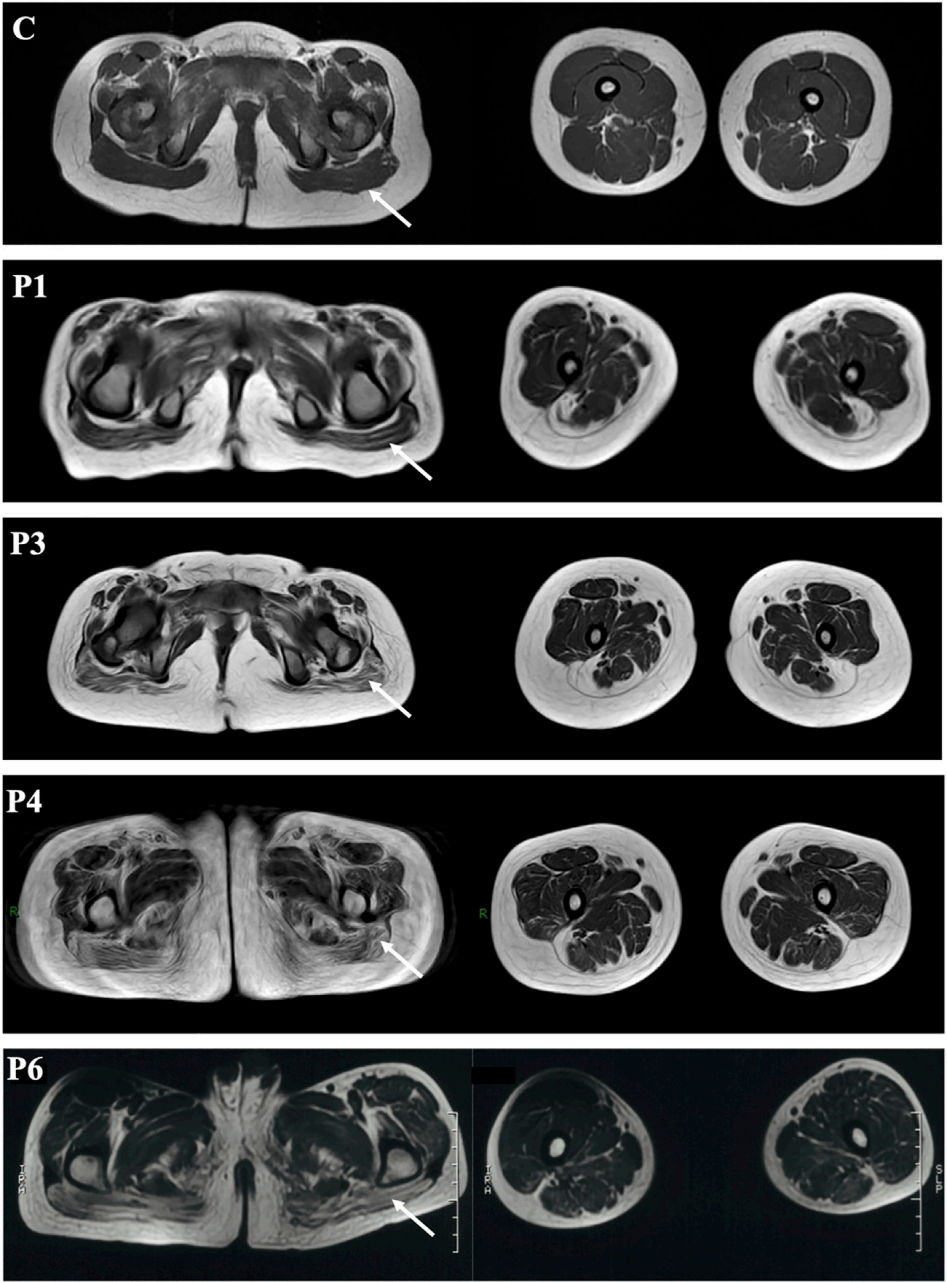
Muscle MRI of RSMD1 patients. Arrows showed the gluteus maximus and thigh. C: control.
